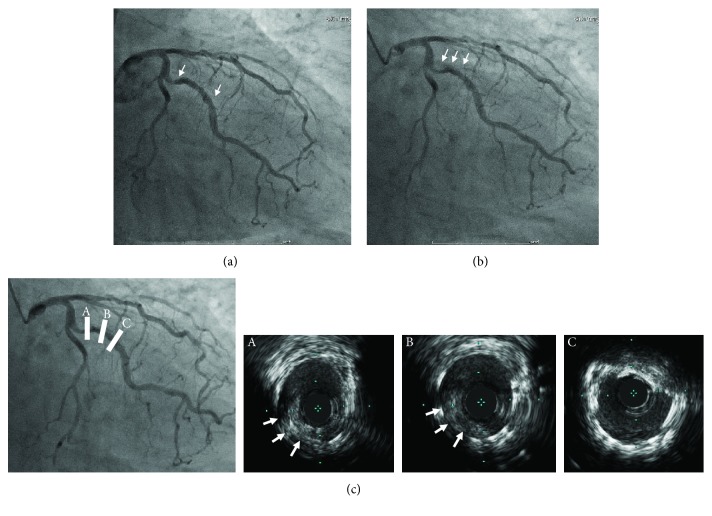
CAG and intravascular ultrasound images during PCI. In the first PCI, a zotarolimus-eluting stent (3.0 × 12 mm) was implanted in #12 (a, between white arrows). Emergent CAG, which was performed 3 hours after the first PCI, revealed massive thrombus in the stent lesion (b, white arrows). The intravascular ultrasound study (c) showed the new lining thrombus (white arrows) in the stent proximal (A and B), whereas not in the distal (C).
